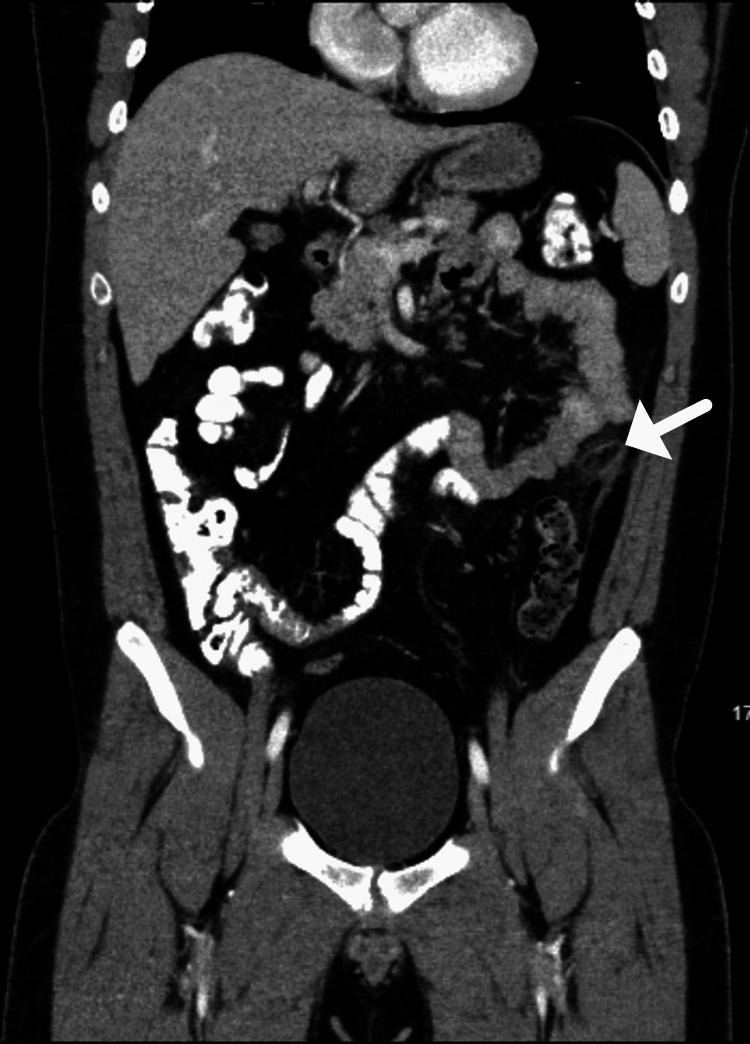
Contrast-enhanced coronal section Abdomen and Pelvis CT scan noting epiploic appendagitis at the mid descending colon.
